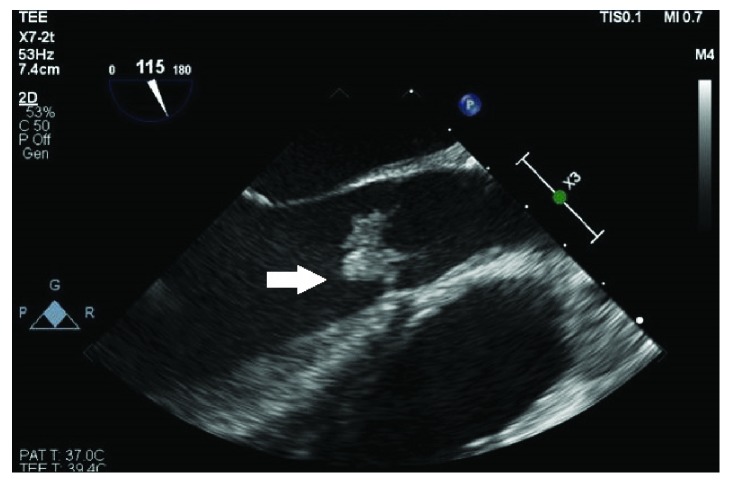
Transesophageal echocardiography revealing large aortic vegetation in longitudinal view (arrow).
